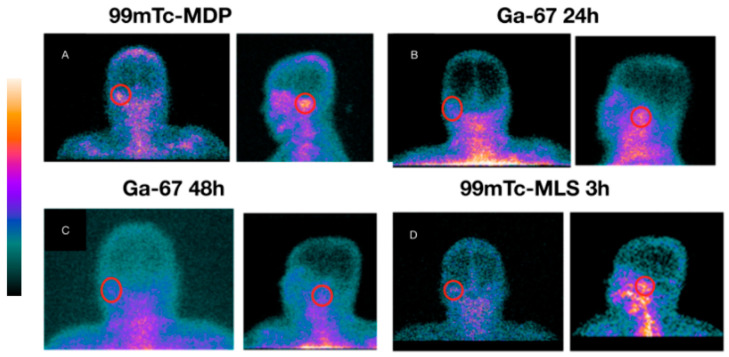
Patient 11. (A) Bone ^99m^Tc-MDP scintigraphy in left mastoid topography (posterior and lateral incidence). (B) ^67^Ga scintigraphy taken 24 h after radiotracer iv. injection showing higher uptake at the region of interest (ROI, red ring). (C) ^67^Ga scintigraphy taken 48 h after radiotracer iv. injection showing a long-lasting higher uptake at the ROI. (D) ^99m^Tc-MLS with a higher uptake 3 h after iv. injection of labeled cells. A skin biopsy showed a chronic nonspecific inflammatory process characterized by granulation tissue with recently formed blood capillaries, fibroblasts, and infiltrated leukocytes at the site.
